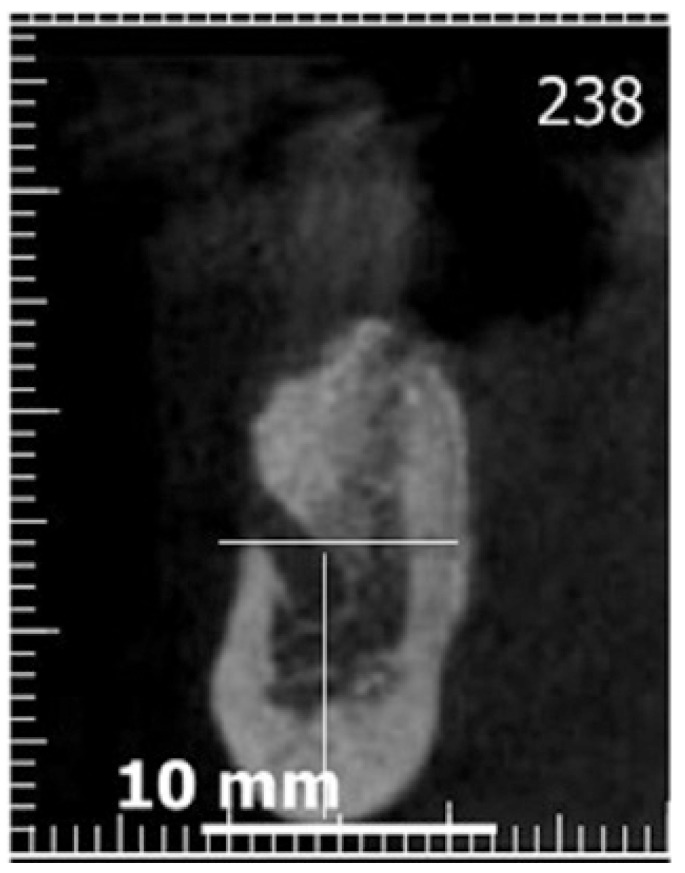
Cross-sectional cut on cone beam computed tomography(CBCT). We observe the technique used for the measurement of the distance between the mental foramen and the lower border of the mandible.
